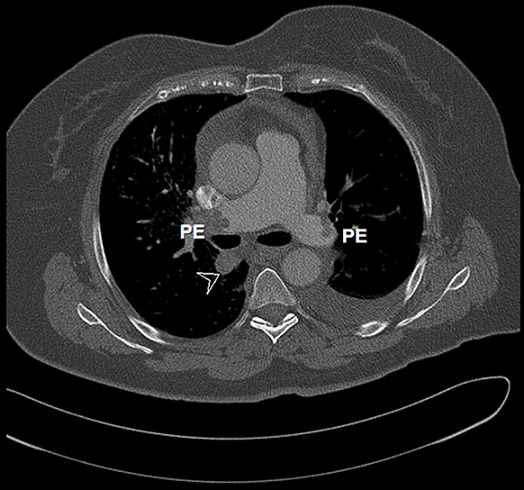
A chest computed tomography scan showing mass lesion in the right lower lung lobe (arrow), and bilateral proximal pulmonary embolism (PE)
